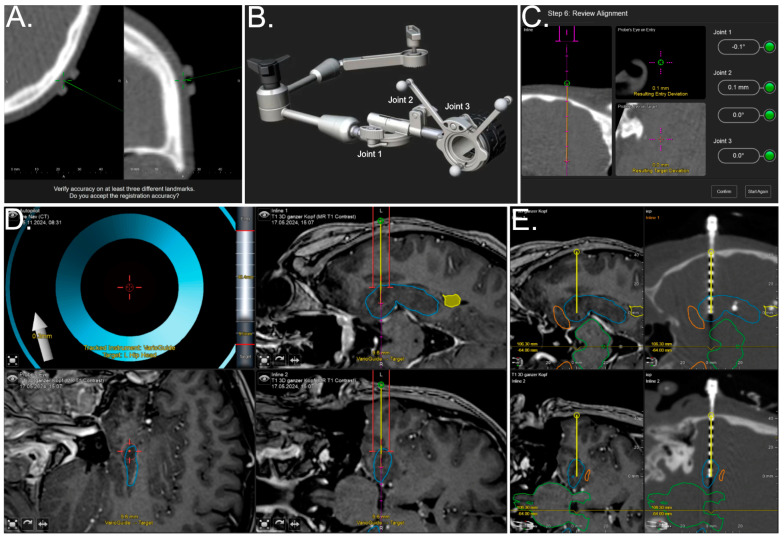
After automated iCT-based registration, the accuracy of registration is inspected and verified using skin-adhesive markers placed on the patient’s head within the scan region (A). Following the initialization of the VarioGuide with all joints set to zero (B), the VarioGuide is adjusted joint by joint to fit the preplanned trajectory (C). The navigational screen displays the alignment of the VarioGuide (red lines, projected into the navigational field using a virtual extension) around the planned trajectory (yellow), which is intended to be placed in the left hippocampal head outlined in blue (D). Intraoperative analysis of trajectory placement accuracy utilizing rigidly fused preoperative MRI (left) and iCT (right) data, with the planned electrode (yellow) visualized over the implanted electrode, indicating a high level of implantation accuracy (E).
